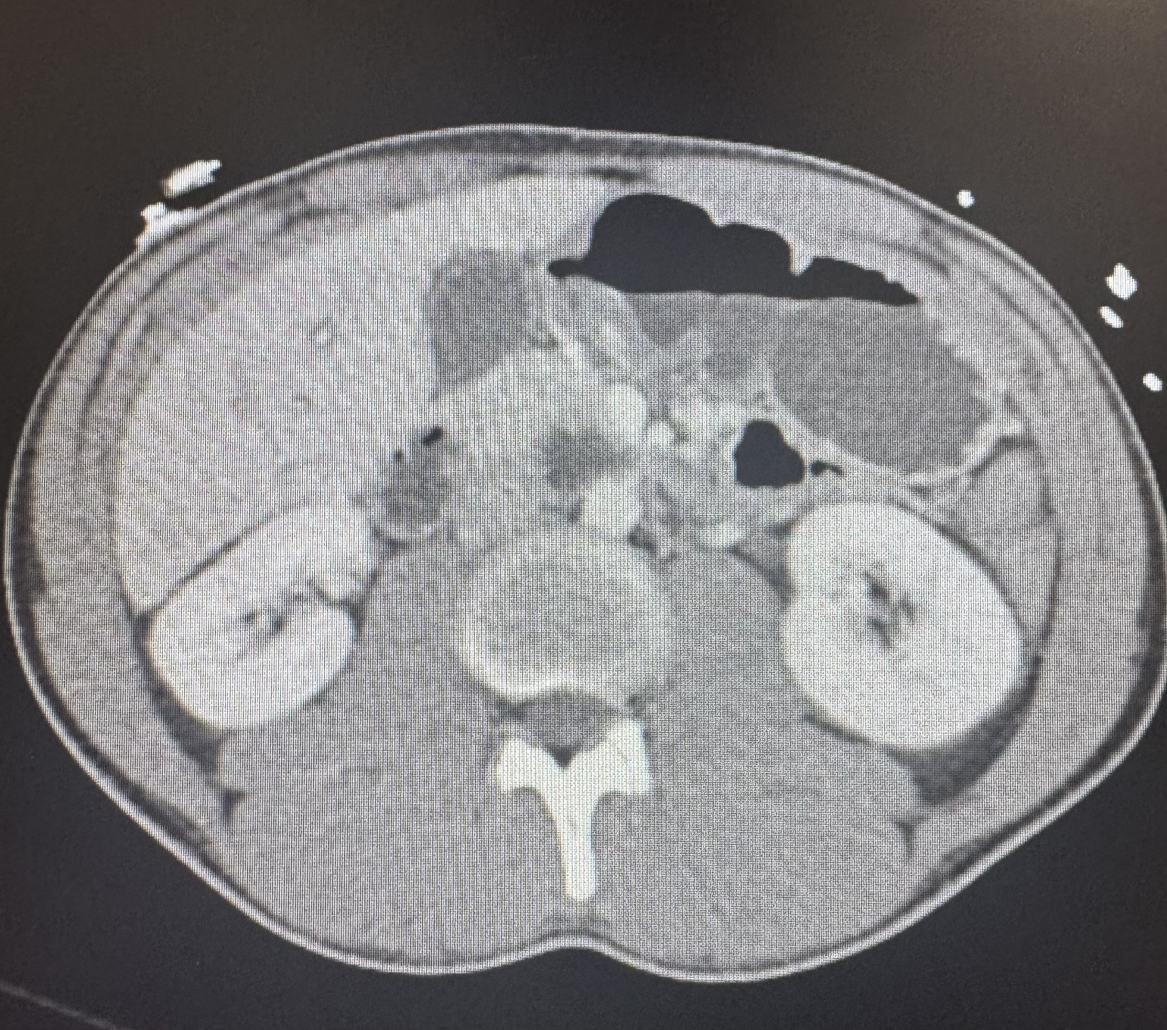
CT abdomen/pelvis with IV contrast CT scan of the patient's abdomen showing no evidence of intra-abdominal infection or inflammation CT: computed tomography
